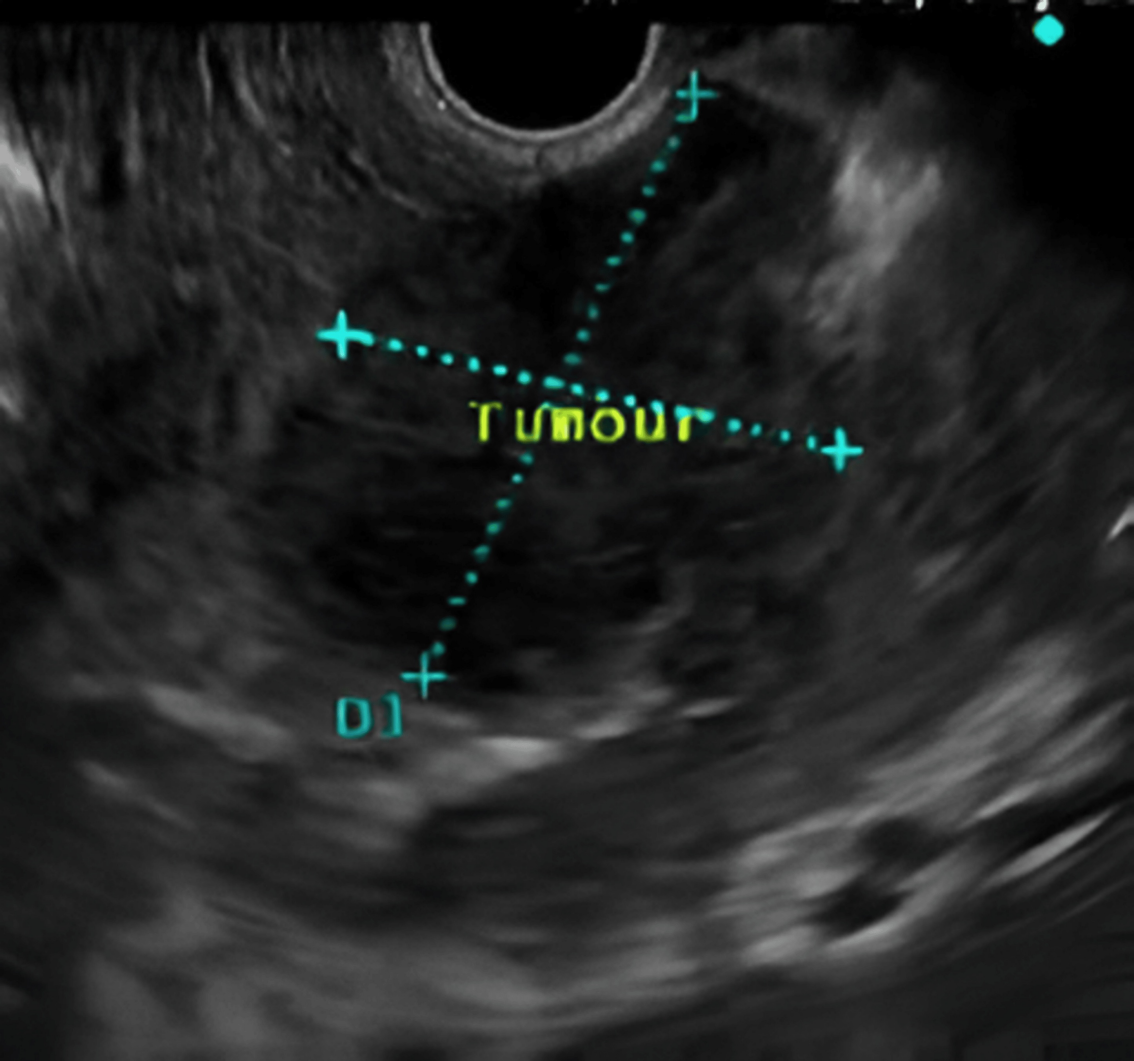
Endoscopic ultrasound showing the hypoechoic lesion
